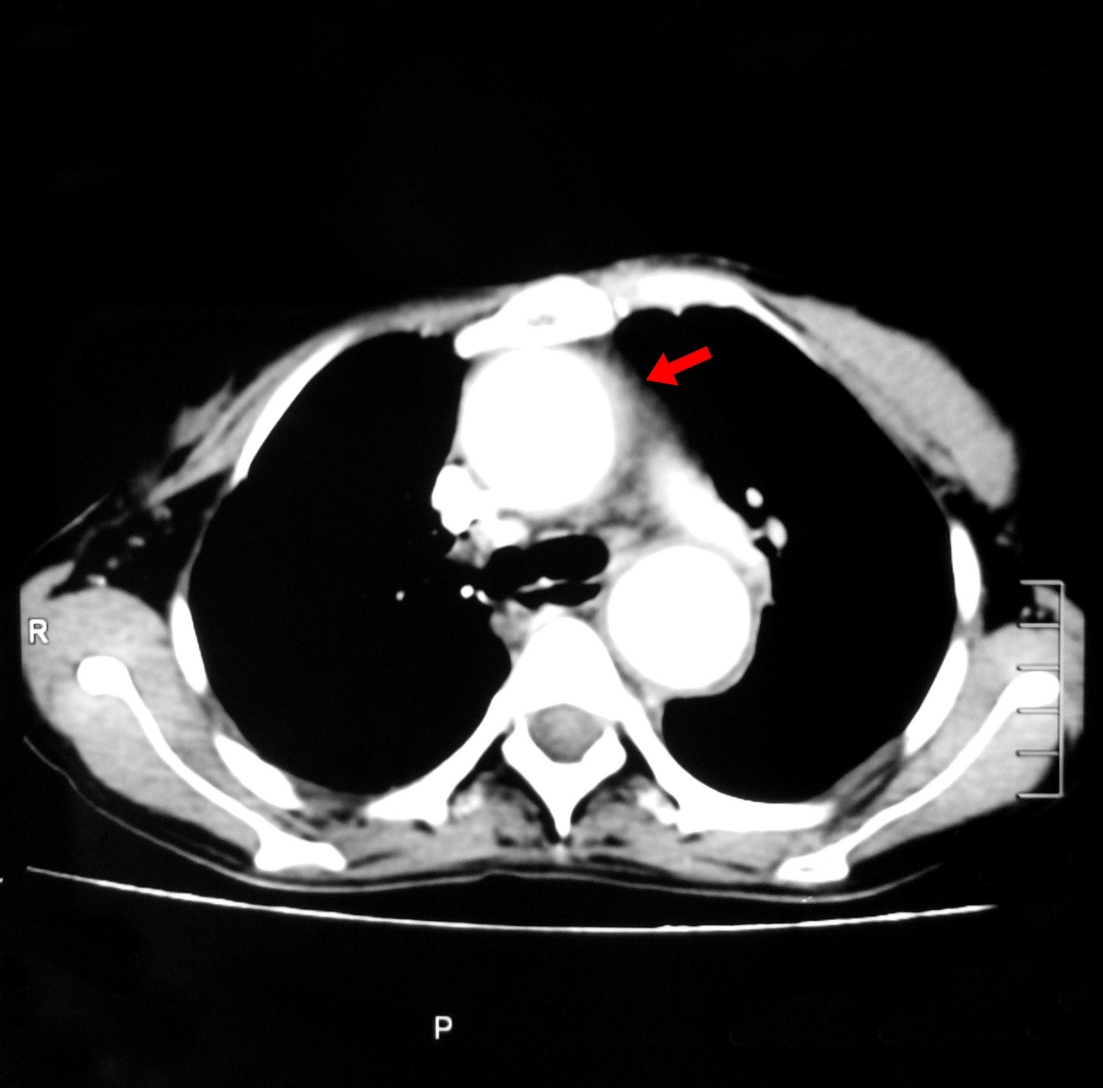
Computed tomography (CT) scan with contrast of chest showing dilation of aorta.
